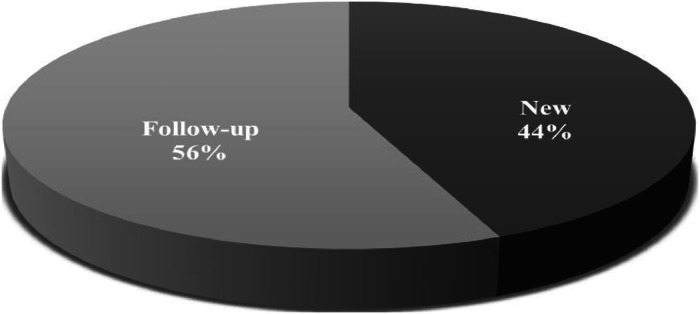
Distribution of the patient cohort by case type, showing 44 % newly diagnosed cases and 56 % follow-up cases.•The ``Spleen_Maximum_Dimension'' attribute represents the longest measurable axis of the spleen in centimeters, extracted from CT scans. This measurement was taken from axial, coronal, or sagittal planes—whichever was the largest. It serves as a quantitative biomarker for detecting splenomegaly and assessing its severity.•The ``Splenomegaly_Status'' attribute determines whether a patient has spleen enlargement based on predefined criteria. Assessing this is somewhat subjective, as there is no universally agreed-upon standard. For example, one study [9] classifies a craniocaudal spleen length of up to 12 cm as normal and values between 12 and 20 cm as indicative of splenomegaly. Other studies define splenomegaly using a craniocaudal length exceeding 9.5 cm and width greater than 10.6 cm on CT imaging [8,10]. In this dataset, the Spleen_Maximum_Dimension was adopted [11], applying a 14-cm threshold to identify cases of splenomegaly. This cut-off reflects the radiological assessment practices followed at King Abdullah University Hospital. Fig. 5 depicts the distribution of splenomegaly cases versus non-splenomegaly cases across genders.
